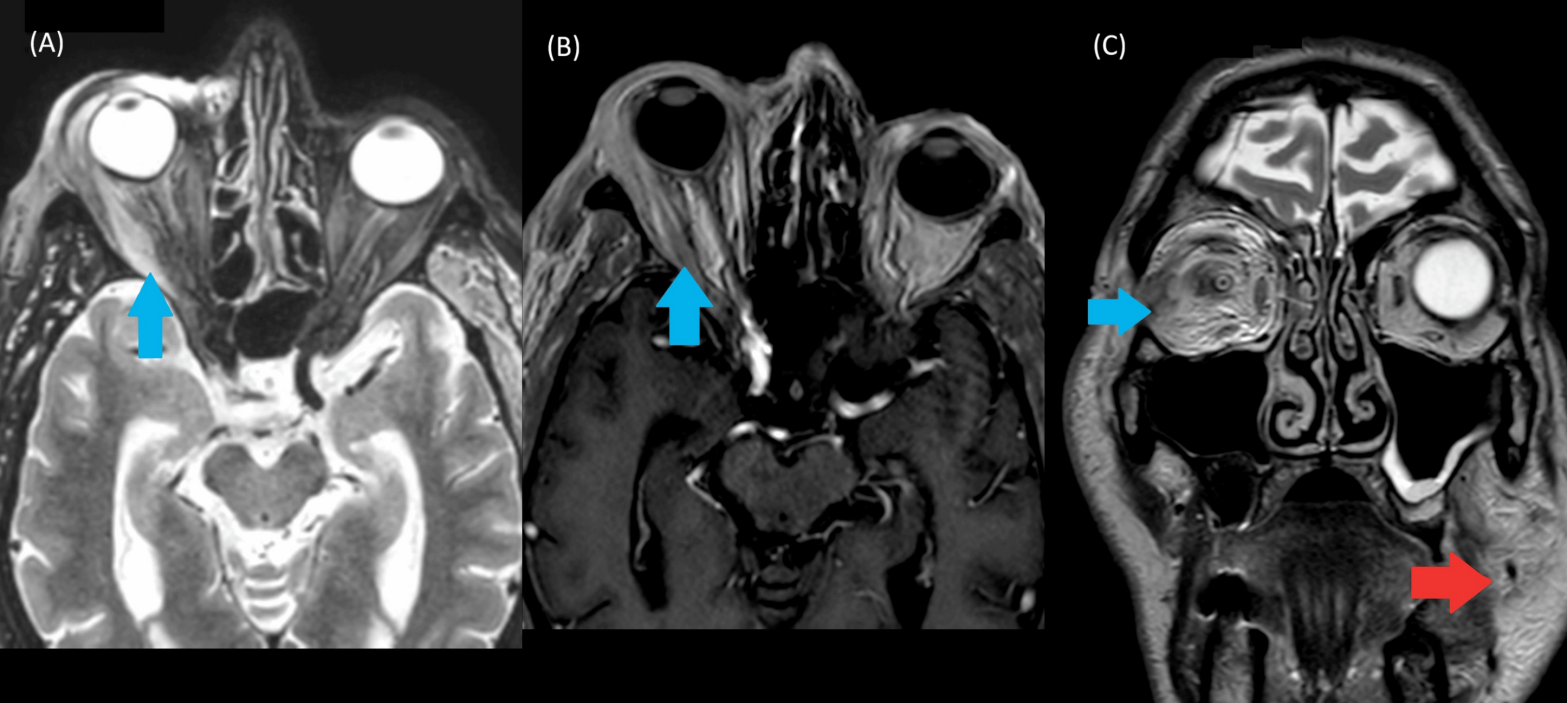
Cerebral and orbital MRI (A) Orbital MRI (axial T2-weighted and STIR-long TE sequences) demonstrating diffuse hyperintense signal and enlargement of the extraocular muscles (blue arrow), consistent with active inflammatory edema. (B) Orbital MRI (axial T1 post-gadolinium) showing diffuse enhancement and mild enlargement of the extraocular muscles (blue arrow), compatible with inflammatory myositis. (C) Cerebral MRI (coronal STIR) showing diffuse hyperintensity and enlargement of the extraocular muscles (blue arrow), reflecting active inflammatory edema, with concomitant edema of the masseter muscles (red arrow) MRI: magnetic resonance imaging; STIR: short tau inversion recovery
